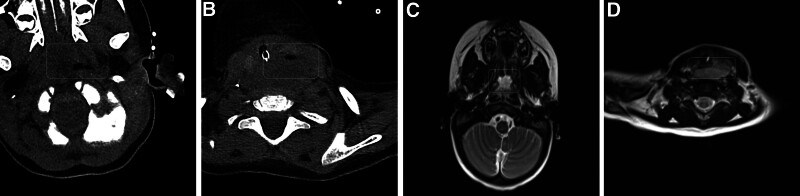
Cervical imaging demonstrating retropharyngeal abscess complications. (A, B) Contrast-enhanced CT: Large cystic lesion compressing the oropharynx, trachea, and left carotid sheath. (C, D) MRI with DWI: Esophageal fistula in the left posterior cervical esophageal wall with an air-fluid level. Maximum compression at the laryngeal-oropharyngeal junction. CT = computed tomography, DWI = diffusion weighted imaging, MRI = magnetic resonance imaging.
